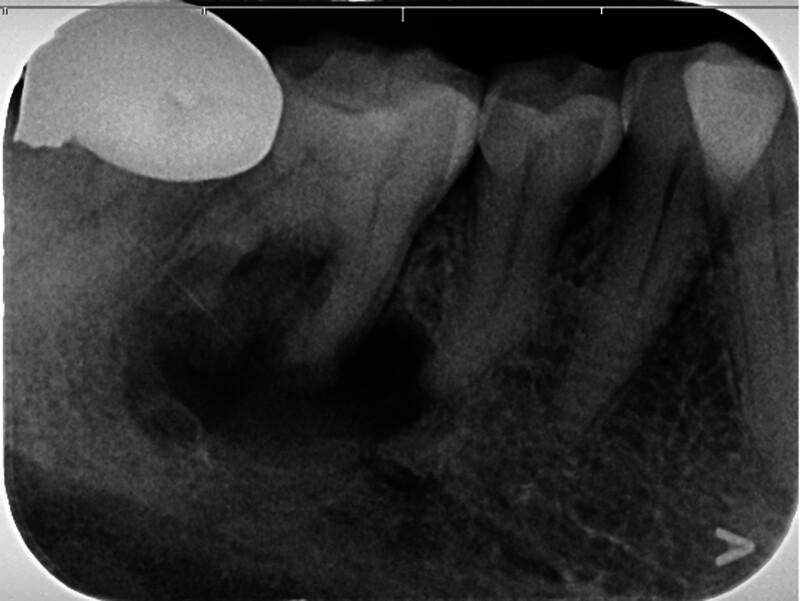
Digital periapical radiograph: a cyst-like low-density shadow is shown in the periapical areas of the right mandibular first molar and the second premolar. The density is uneven and the boundary is clear. There is a slight external root resorption at the root apex of the first molar (the lesion is significantly larger compared with that in February 2017).
